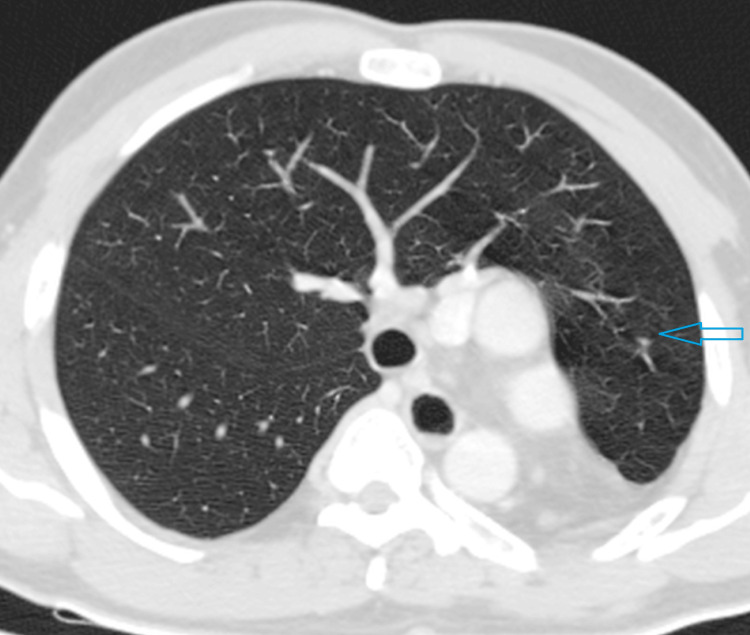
CT scan of the chest in the lung window reveals a complete absence of the left lung with compensatory hyperinflation and transmediastinal herniation of the right lung CT: computed tomography
